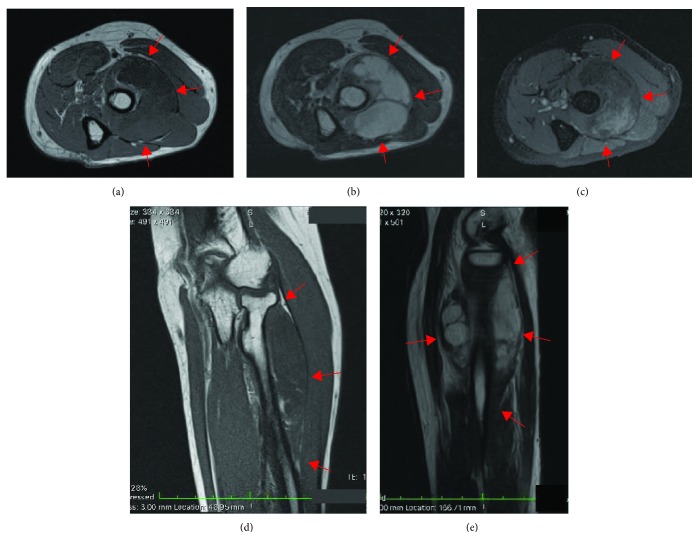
Magnetic resonance imaging (MRI) showed a lobulated multiseptated lesion seen within left supinator muscle as delineated by the red arrows, which showed a hyperintense signal on T2-weighted images (T2WI) (b, e) and hypointense on T1-weighted images (T1WI) (a, d), with peripheral enhancement post contrast (c).
